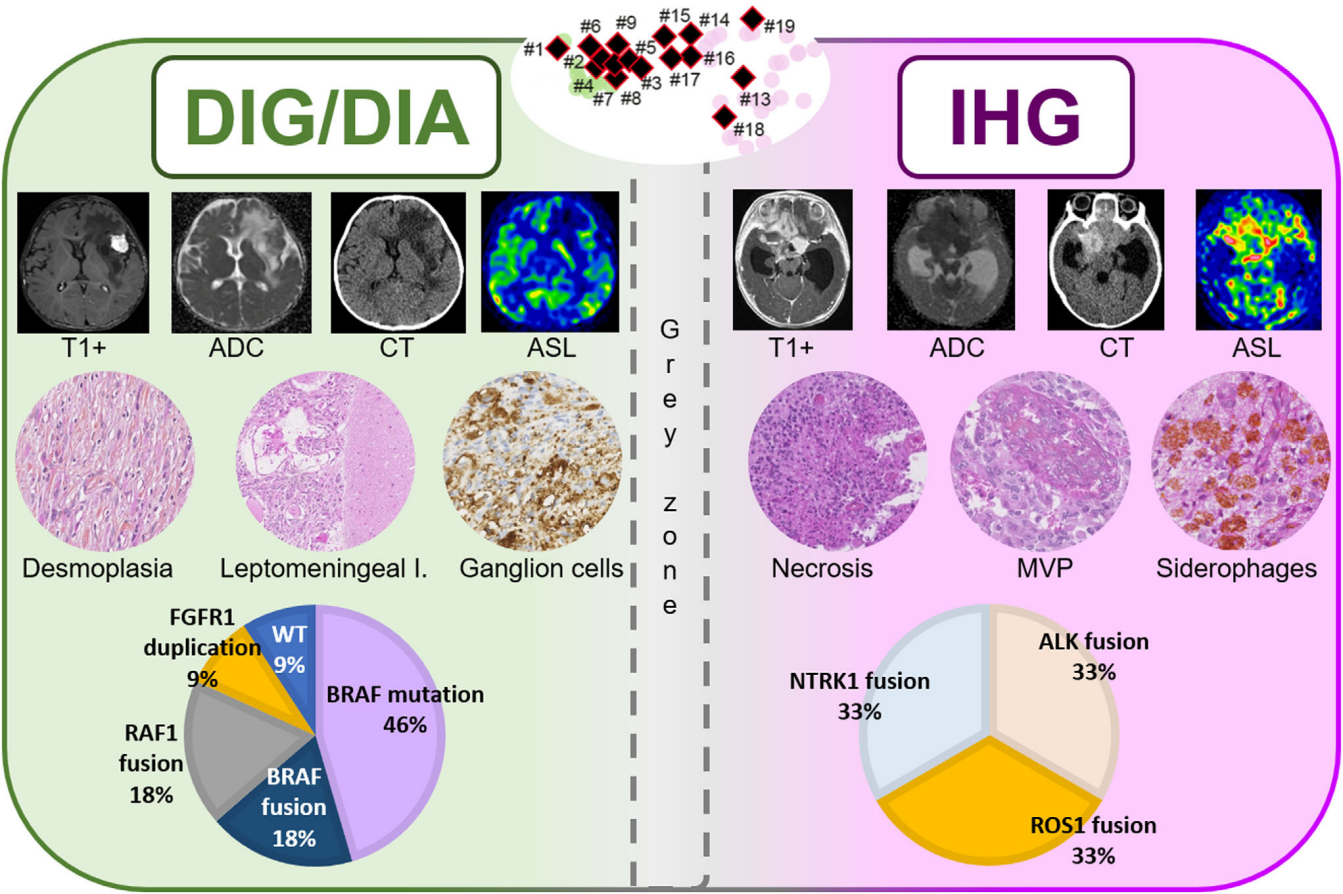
Summary of desmoplastic infantile gangliogliomas/astrocytomas and infant‐type hemispheric gliomas main characteristics. Both DIG/DIA and IHG displayed avid contrast enhancement, but cysts walls of DIG/DIA did not enhance, in comparison with enhancing cysts walls of IHG. Diffusion restriction on ADC maps was moderate in DIAG/DIA patients and strongly restricted in IHG. IHG had frequent bleeding on CT, and not DIA/DIG. Perfusion values using ASL were moderate in DIG/DIA patients and high in IHG. DIG/DIA presents desmoplasia, infiltration of the leptomeninges, and a neuronal differentiation whereas IHG showed obvious signs of malignancy (necrosis and MVP), and siderophages (suggesting a past hemorrhage). Molecular alterations are distinct between DIG/DIA (showing MAPK genes alterations) and IHG (showing RTK gene fusions). DNA‐methylation profiles of DIG/DIA and IHG are distinct but a grey zone exists with overlapping cases. ADC, diffusion coefficient map; ASL, arterial spin labeling; CT, computerized tomodensitometry; DIG/DIA, desmoplastic infantile agangligliomas/astrocytomas; I, involvement; IHG, infant‐type hemispheric gliomas; MAPK, mitogen‐activated protein kinase; MVP, microvascular proliferation; RTK, receptor tyrosine kinase; WT, wildtype.
